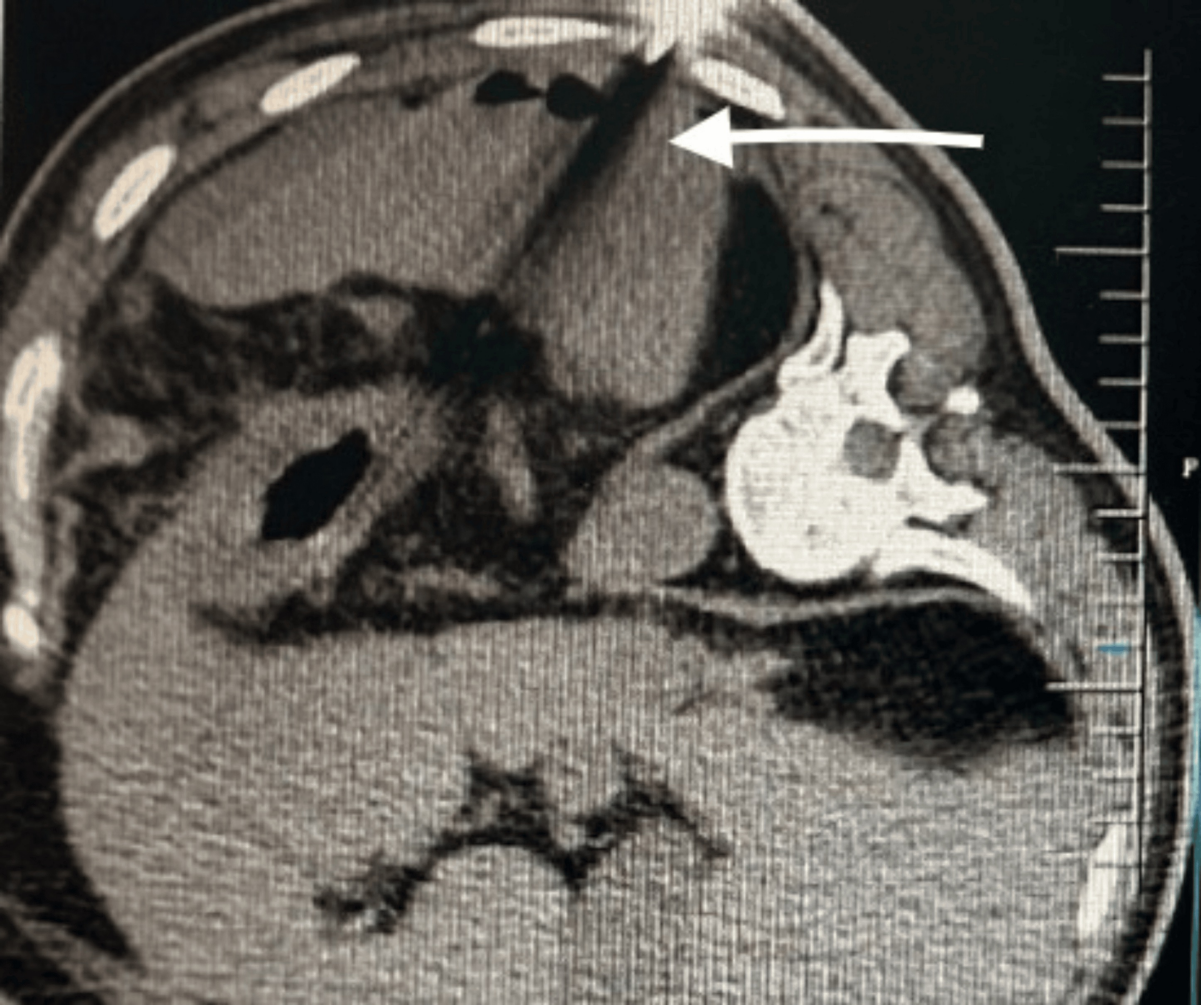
Insertion of a pigtail in a splenic abscess under CT guidance
